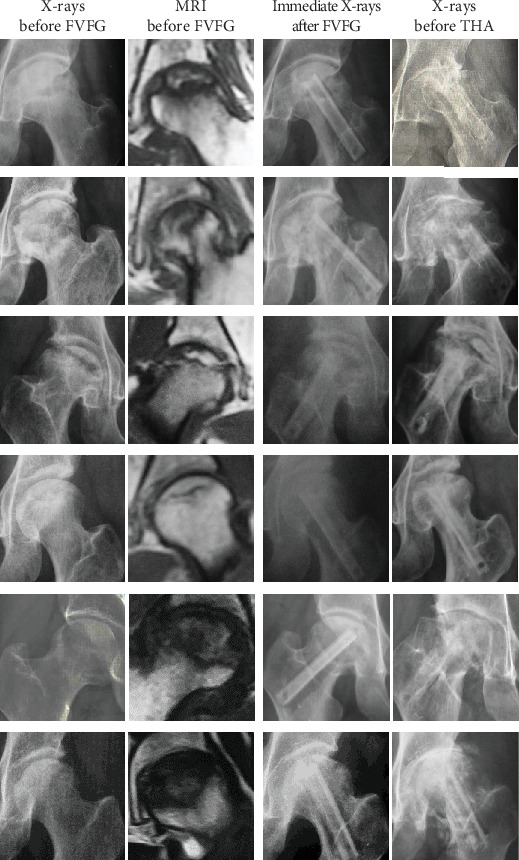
Six failure cases' radiographs (X-rays and MRI before FVFG, immediate X-rays after FVFG, and X-ray before THA).
